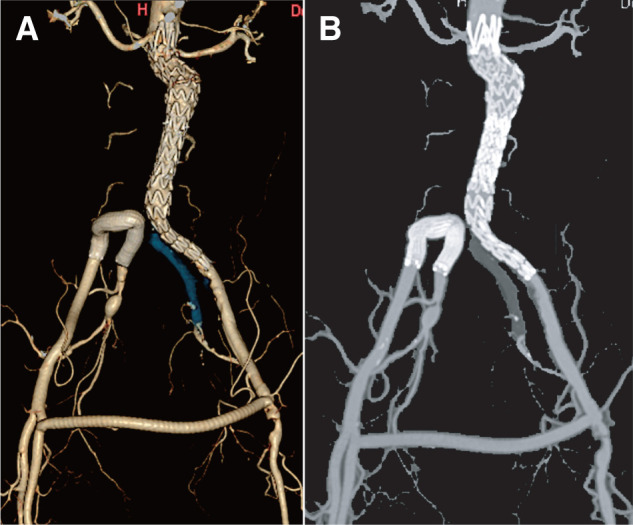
Postoperative computed tomography findings. (A) Three-dimensional computed tomography scan showing patency of the covered stent reconstruction and femorofemoral bypass with complete exclusion of the aneurysm sac. (B) Axial computed tomography image confirming patency of both the covered stent and femorofemoral bypass graft without evidence of endoleaks.
